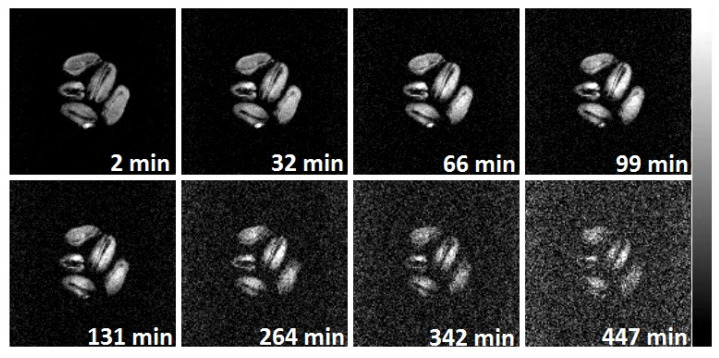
MRI images of wheat grains during natural air drying at 30 °C. The time elapsed from the beginning of the drying process is indicated. Higher signal intensity corresponds to higher moisture level.
